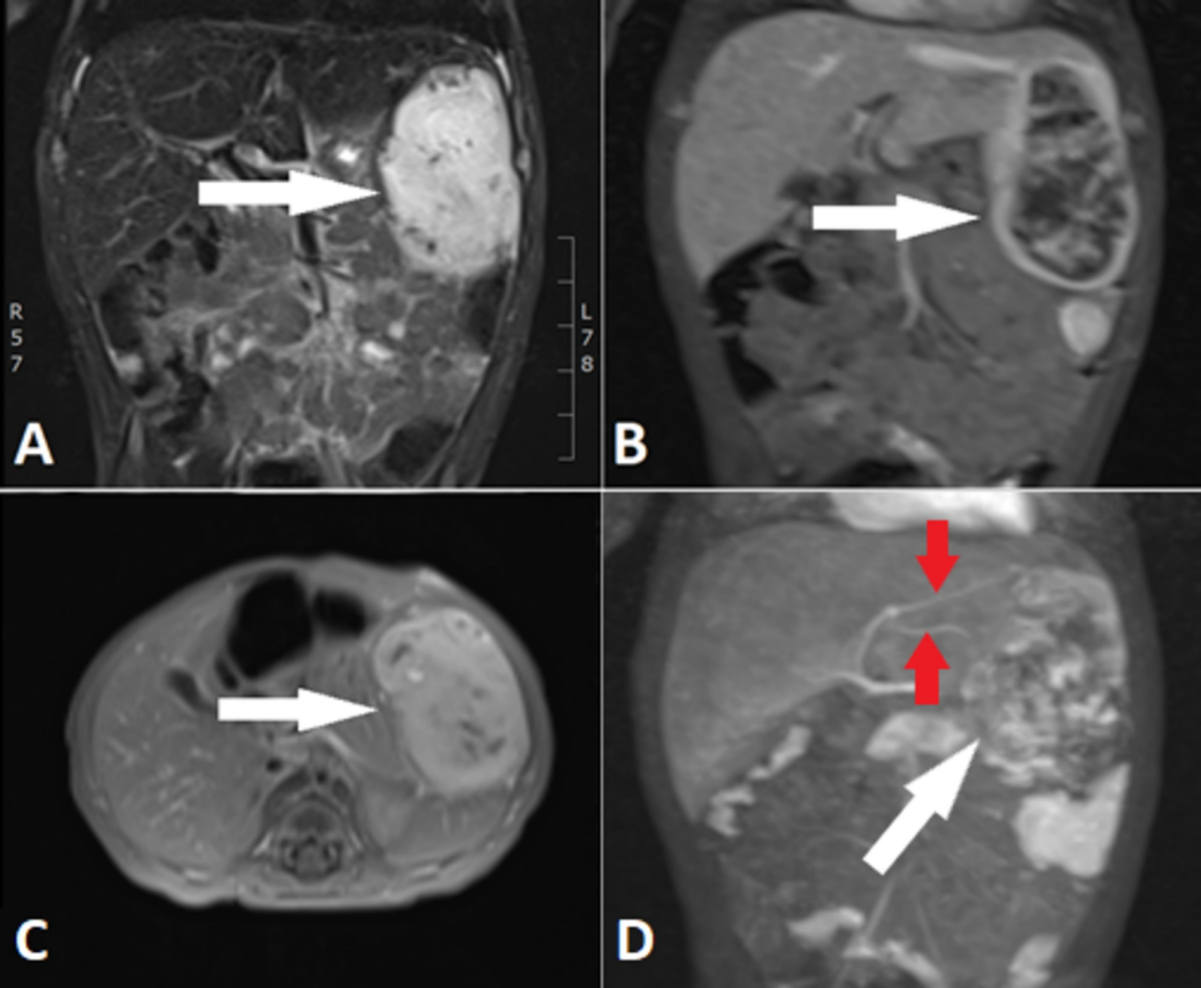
Abdominal MRI An abdominal MRI showing a focal hepatic lesion (white arrow) in different phases and sequences. (A) A coronal T2 fat sat sequence showing a heterogeneous hyperintensive focal lesion rising from the left hepatic lobe. (B) Coronal T1 fat sat vibe contrast medium showing a peripherally enhancing lesion with hypointense central part in the arterial phase. (C) Oblique T1 fat sat late contrast-enhanced venous phase showing a lesion completely filled with contrast medium with same hypointense necrotic areas. (D) Maximum intensity projection (MIP) reconstruction of arterial phase showing two feeding arteries (red arrows).
